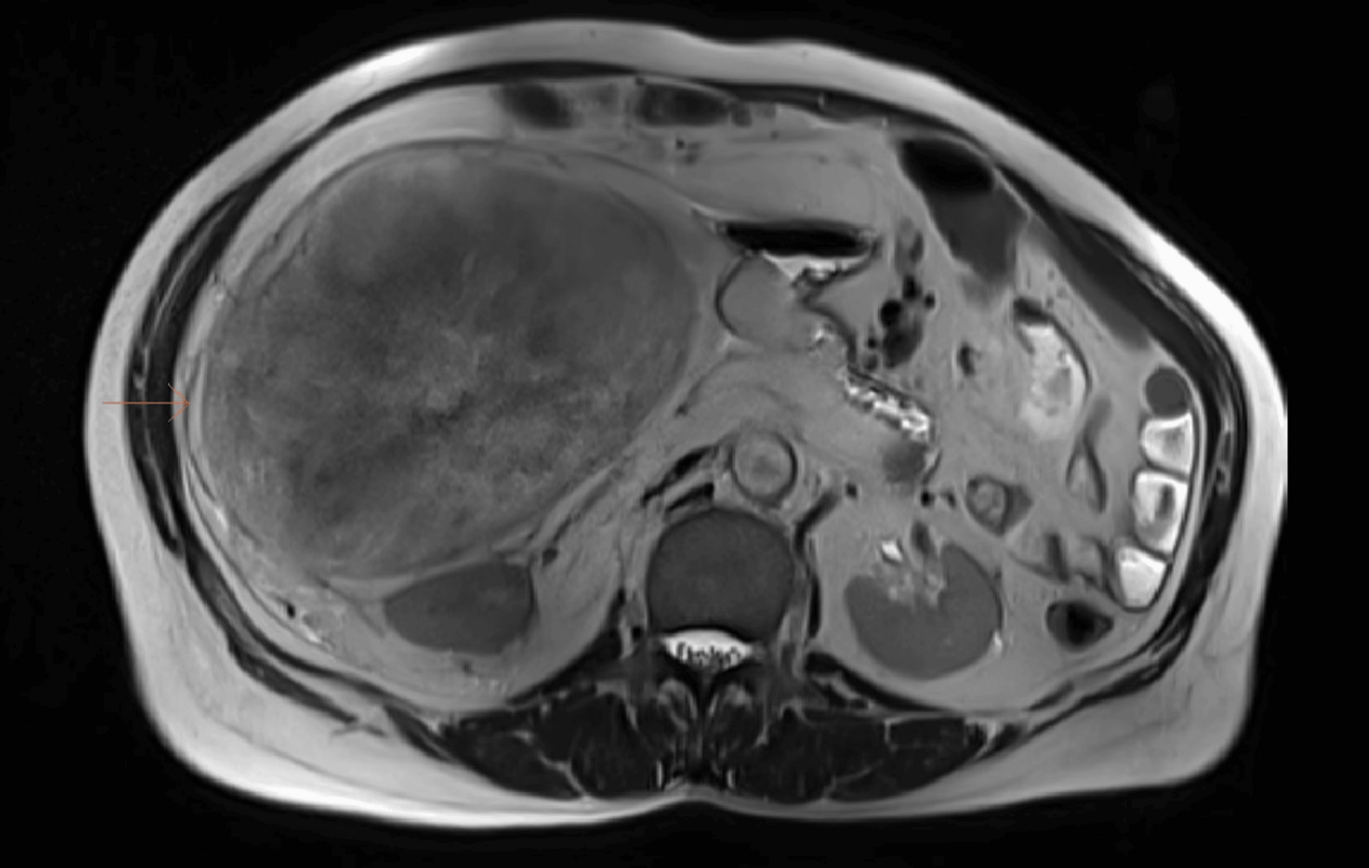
MRI T2WI axial section showing retroperitoneal mass. T2WI, T2-weighted imaging.
